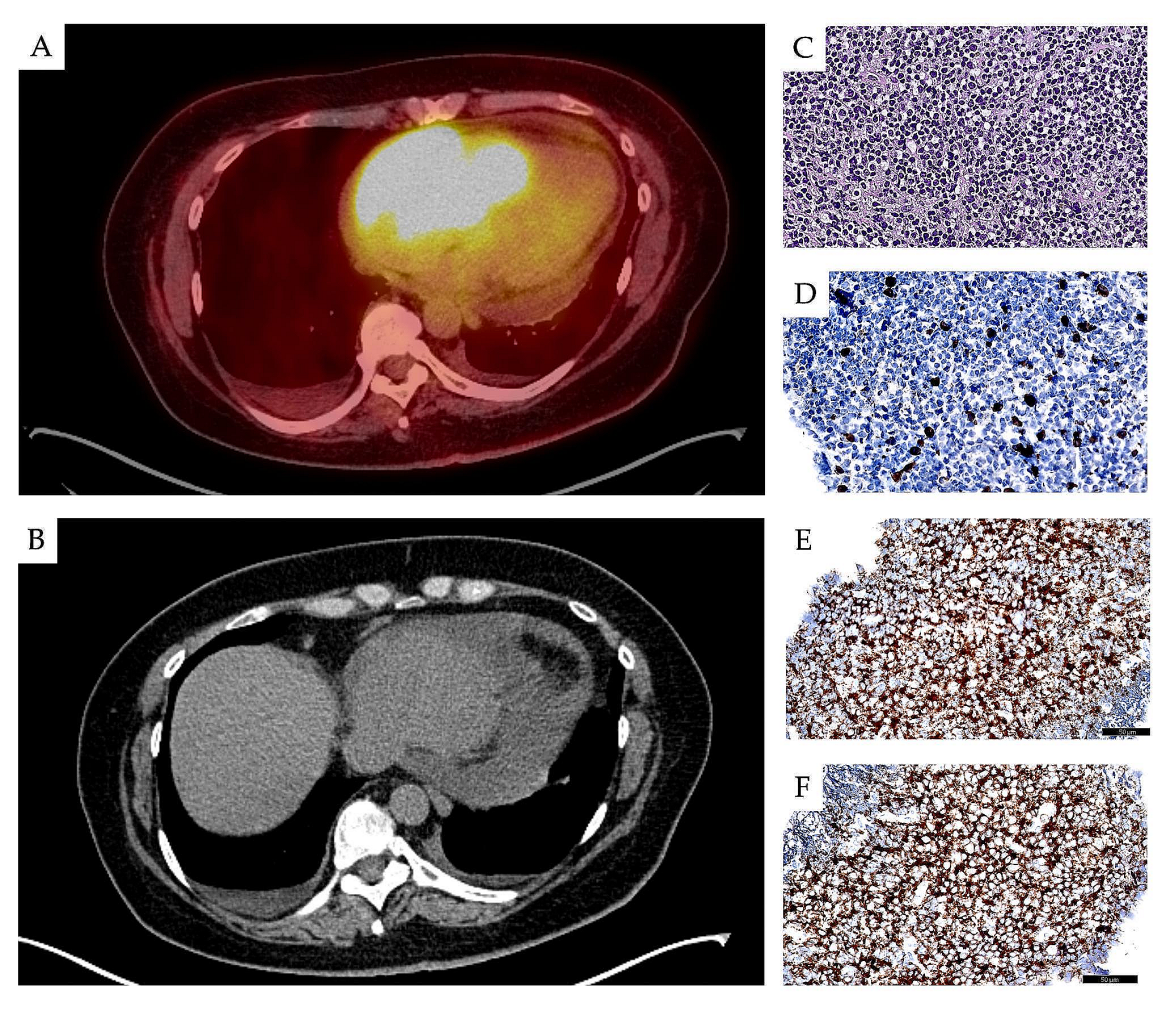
A PET/CT (A) and a CT scan of a CLL/SLL heart infiltration. Infiltration of CLL/SLL in intracardiac biopsy (digitalized, Philips IntelliSite scanner) of the masses in right ventricular epicardium with cytomorphology of small B-cells (C) immunophenotype showed negativity for CD3 (D) and strong positivity for CD23 (E) and CD5 (F)
